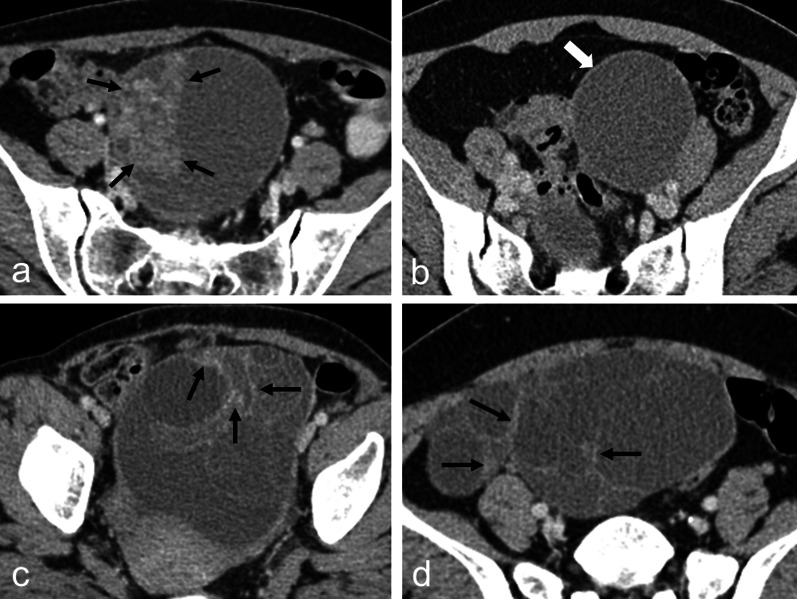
Contrast-enhanced CT images of ovarian tumors that were misclassified by AI model or/and junior radiologists. a A malignant ovarian tumor (clear cell carcinoma) that was predicted to be benign by AI model but malignant by all junior radiologists. The solid portion (arrow) in the tumor is a clue for malignancy in radiological evaluation. b A benign ovarian tumor (endometrioma) that was predicted to be malignant by AI model but benign by all junior radiologists. There was no solid portion, mural nodule, or thick septa to indicate malignancy in radiological evaluation. c A benign ovarian tumor (mucinous cystadenoma) that was predicted to be malignant by both AI model and all junior radiologists. d A benign ovarian tumor (mucinous cystadenoma) that was predicted to be malignant by all junior radiologists but benign by AI model. Thick septa (arrow) in c and d raised the suspicion of malignancy in radiological evaluation
